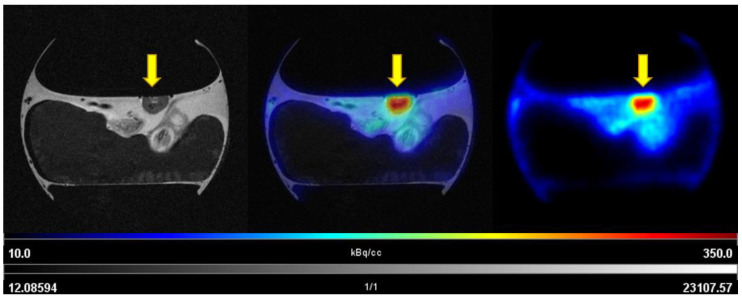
Representative images of 2-[^18^F]FDG uptake into HCT116 CAM-xenograft. Uptake of 2-[^18^F]FDG into the HCT116 xenograft after injection of 4.21 MBq 2-[^18^F]FDG into a CAM-vessel on EDD18. PET imaging was performed 60 min p.i. Left: T2-weighted TurboRARE image, right: PET, middle: fused PET and MR (axial planes are shown).
